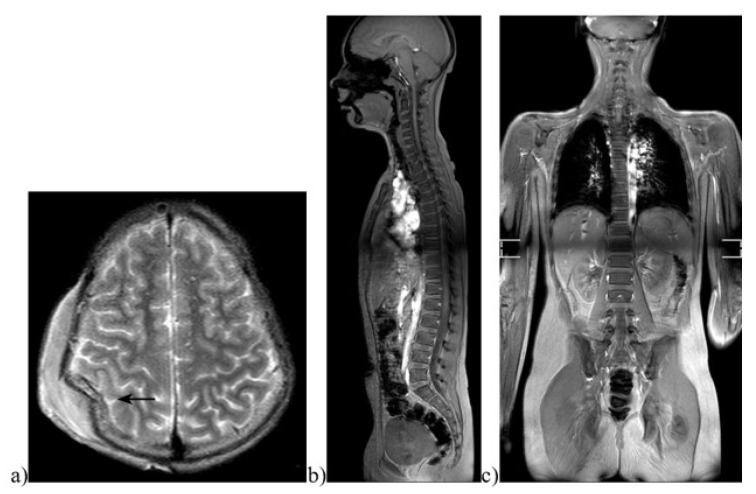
Images of a pediatric whole-body magnetic resonance tomography (WBMR). Example of a 12-year-old boy who was hit by a car as a pedestrian. MRI images showing (a) a large compression fracture and subgaleal hemorrhage on axial TSE T2w imaging (marked by an arrow). The (b) sagittal STIR and (c) coronal STIR full-body images showed no spinal fracture, parenchymal lacerations, or free fluid indicating further injuries. A standard protocol developed specifically for pediatric whole-body imaging via MRI was used [17].
